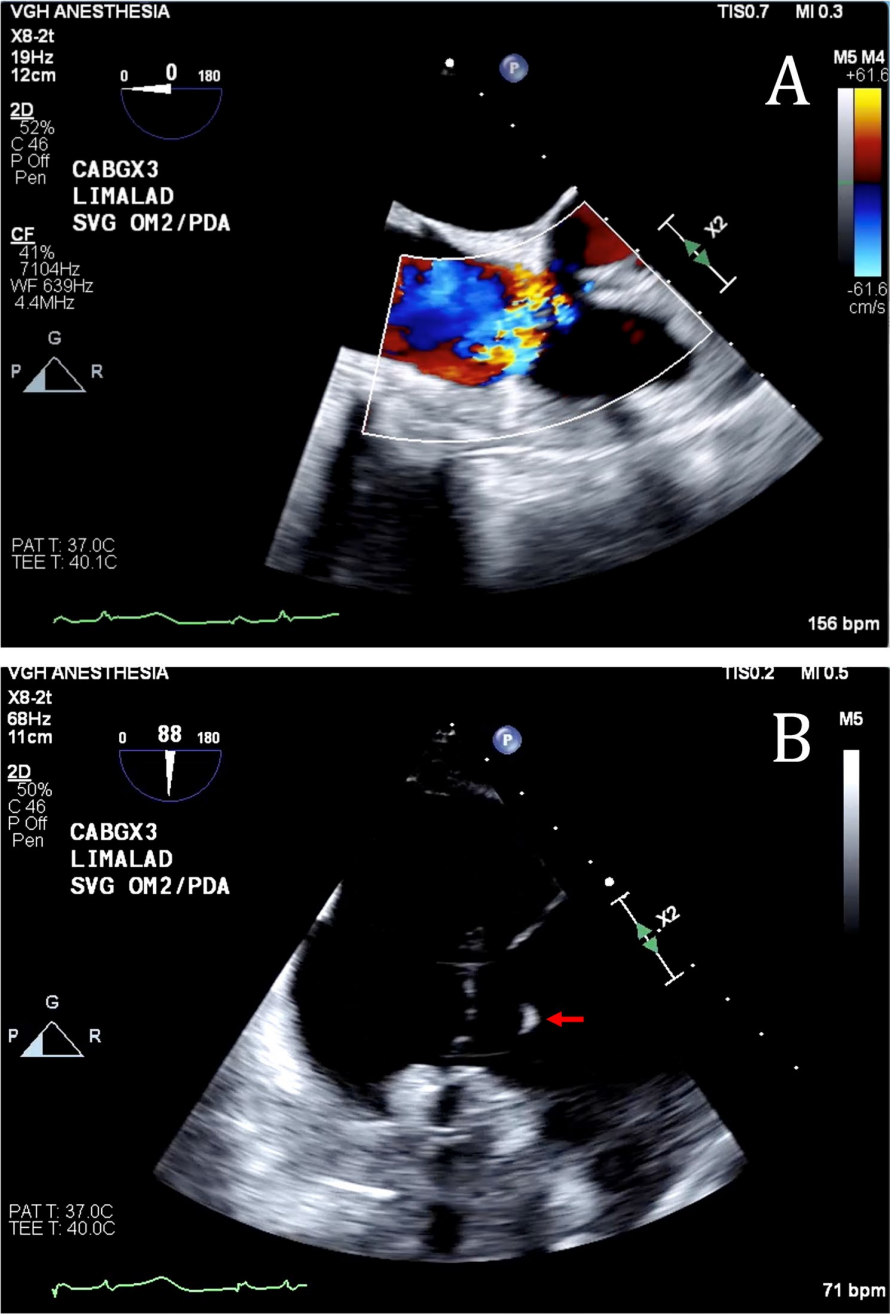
Intraoperative transesophageal echocardiography showing: (A) severe tricuspid regurgitation with an anteriorly directed jet on mid esophageal view and (B) a flail segment (denoted by the red arrow) of the tricuspid valve on a deep transgastric right ventricular view
